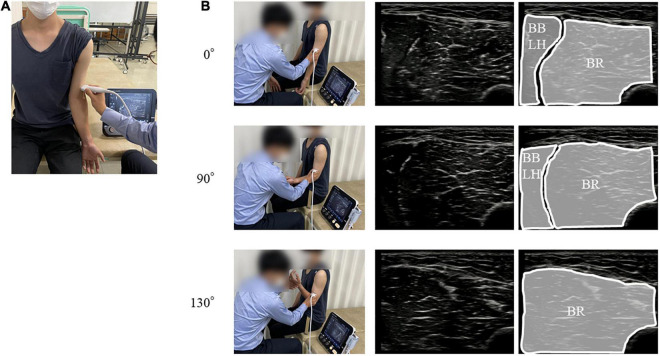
Position and orientation of the ultrasound probe. (A) The position of probe for the BR. (B) The upper, middle, and lower panels represent the position of probe for the BR and ultrasound images of the BR at the 0°, 90°, and 130° elbow flexions, respectively. BR, brachialis muscle.
